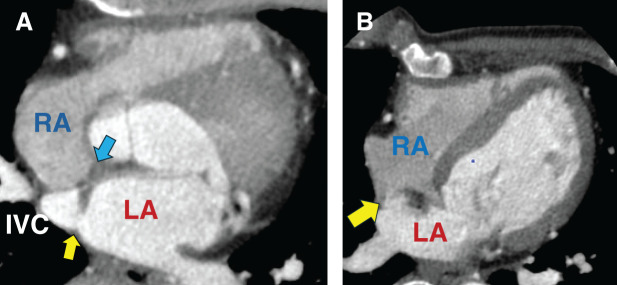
Preoperative CT. (A) Venous blood from the inferior vena cava flows into the left atrium (yellow arrow) instead of the right atrium. Eustachian valve (blue arrow). (B) New ASD (yellow arrow) created in the second surgery. ASD, atrial septal defect; IVC, inferior vena cava; LA, left atrium; RA, right atrium
